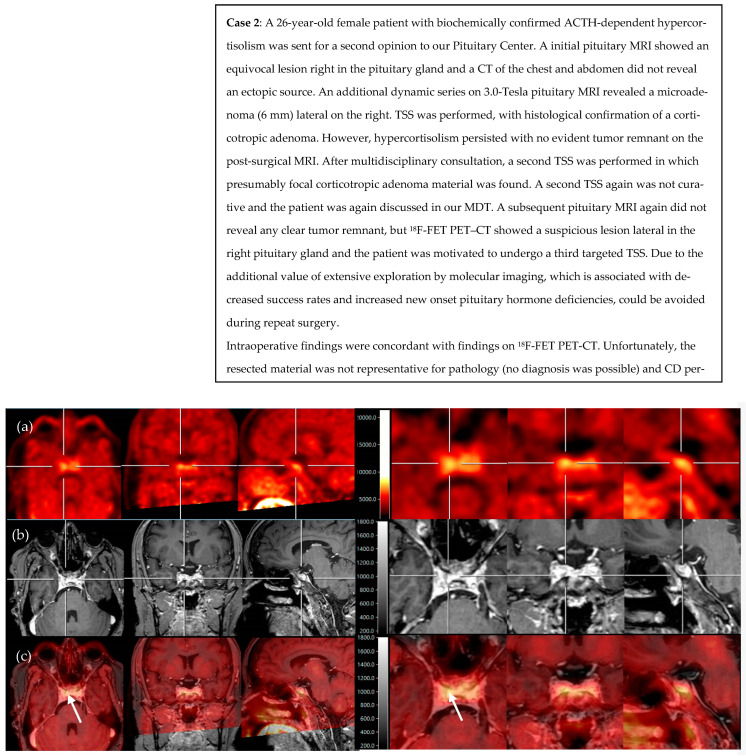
^18^F-FET PET and fusion of PET with post-contrast T1 MR images reveal asymmetrical focal high uptake (in yellow) lateral in the right pituitary, as centered with the crosshair (a–c) and shown with the white arrows (c). (a) axial, coronal, and sagittal view of PET scan after infusion of 192 MBq 18F-FET, (b) axial, coronal, and sagittal view of 3D T1-weighted, post-gadolinium MRI, and (c) axial, coronal, and sagittal view of PET/MRI fusion. Scans on the right side present the same lesion but in more detail.
